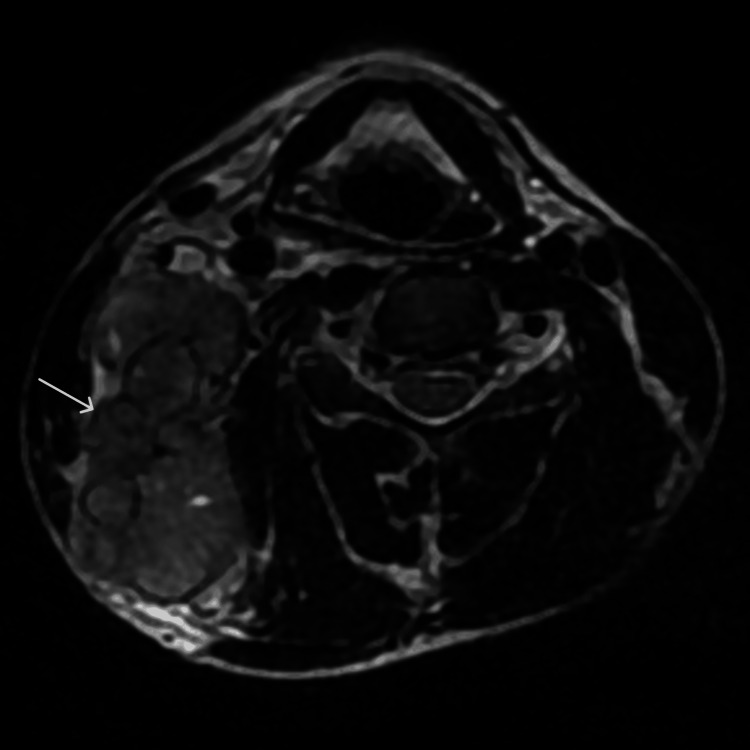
T2-weighted axial images through the neck A conglomerate of multiple enlarged lymph nodes is seen in the left deep cervical region (white arrow).
